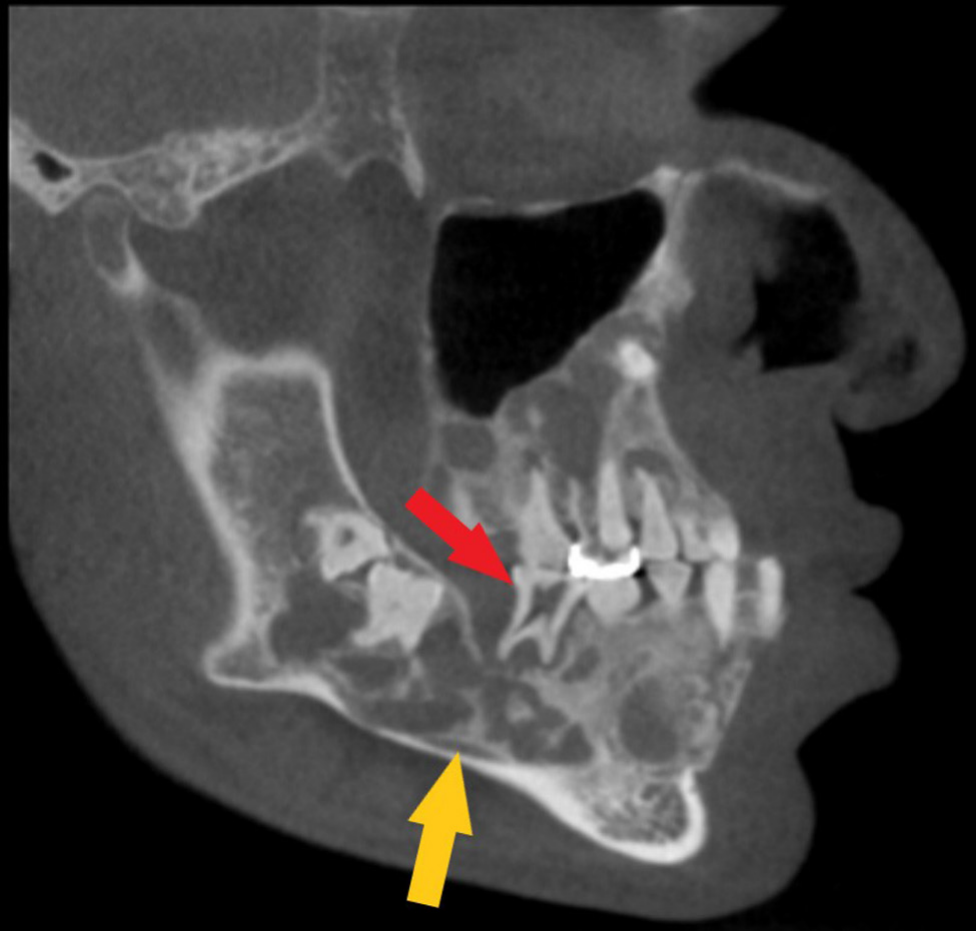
Sagittally reconstructed CBCT of the jaws depicting a mixed-density lesion in the maxilla and mandible containing teeth. There is elongated pulp chamber, apically positioned furcation, shortened roots are noted in tooth #19 and molars on right side, consistent with taurodontism (red arrow). Inferiorly displaced left inferior alveolar canal (yellow arrow). CBCT, cone beam CT.
